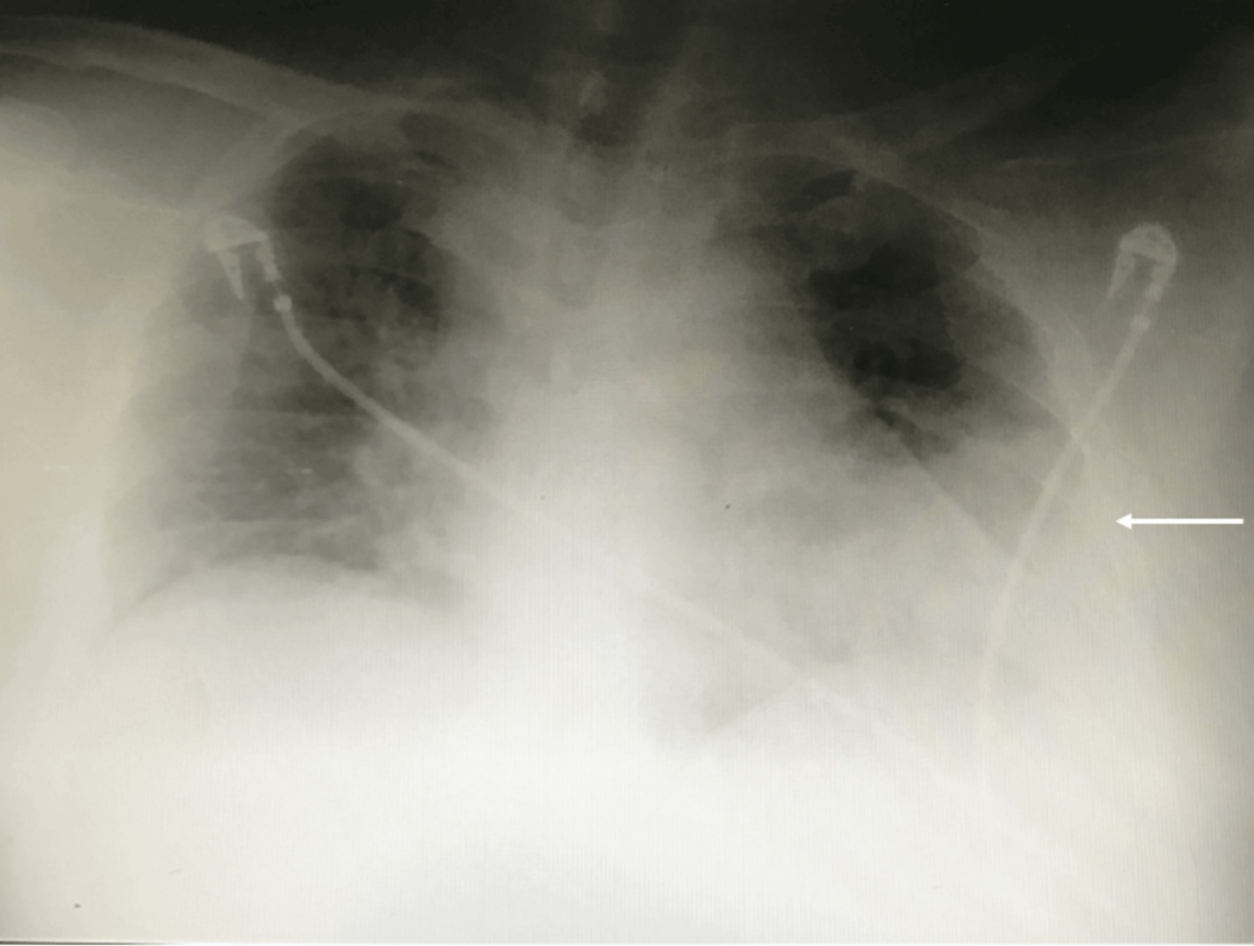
Chest radiograph showing left-sided consolidation (arrow)
